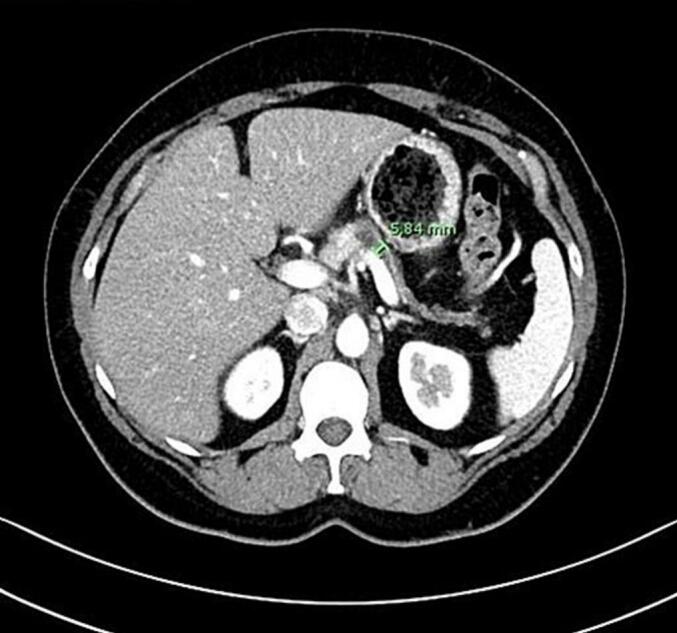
Axial contrast-enhanced computed tomography (CT) scan showing the main pancreatic duct measuring 5.84 mm.
